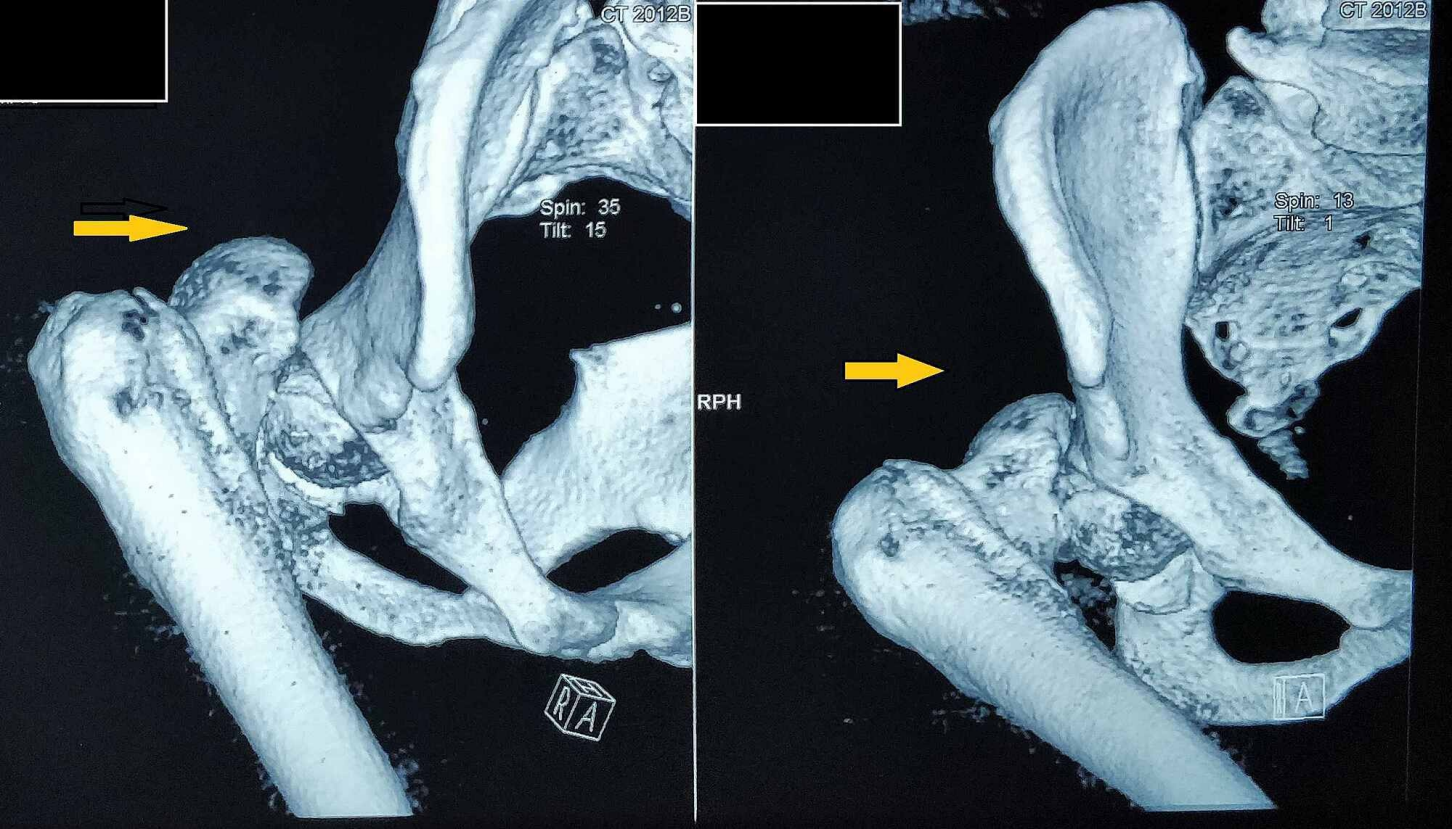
CT scans showing Pipkin fracture-dislocation (arrows) CT: computed tomography
